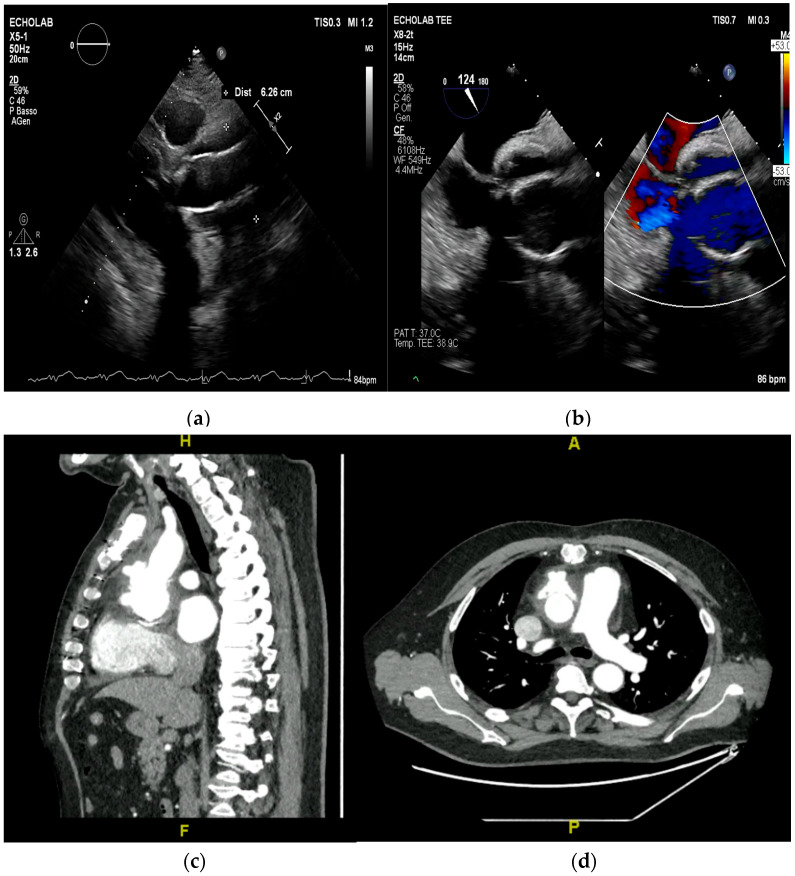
(a–d): These images show a detachment of a valved conduit that was previously implanted for severe aortic regurgitation and aortic root enlargement (a,b). In (c,d) the CT scan further defines the anatomy of the endocarditis, which is particularly useful in presurgical planning. This is an original, de-identified clinical image from our institution. Patient consent for publication was obtained.
